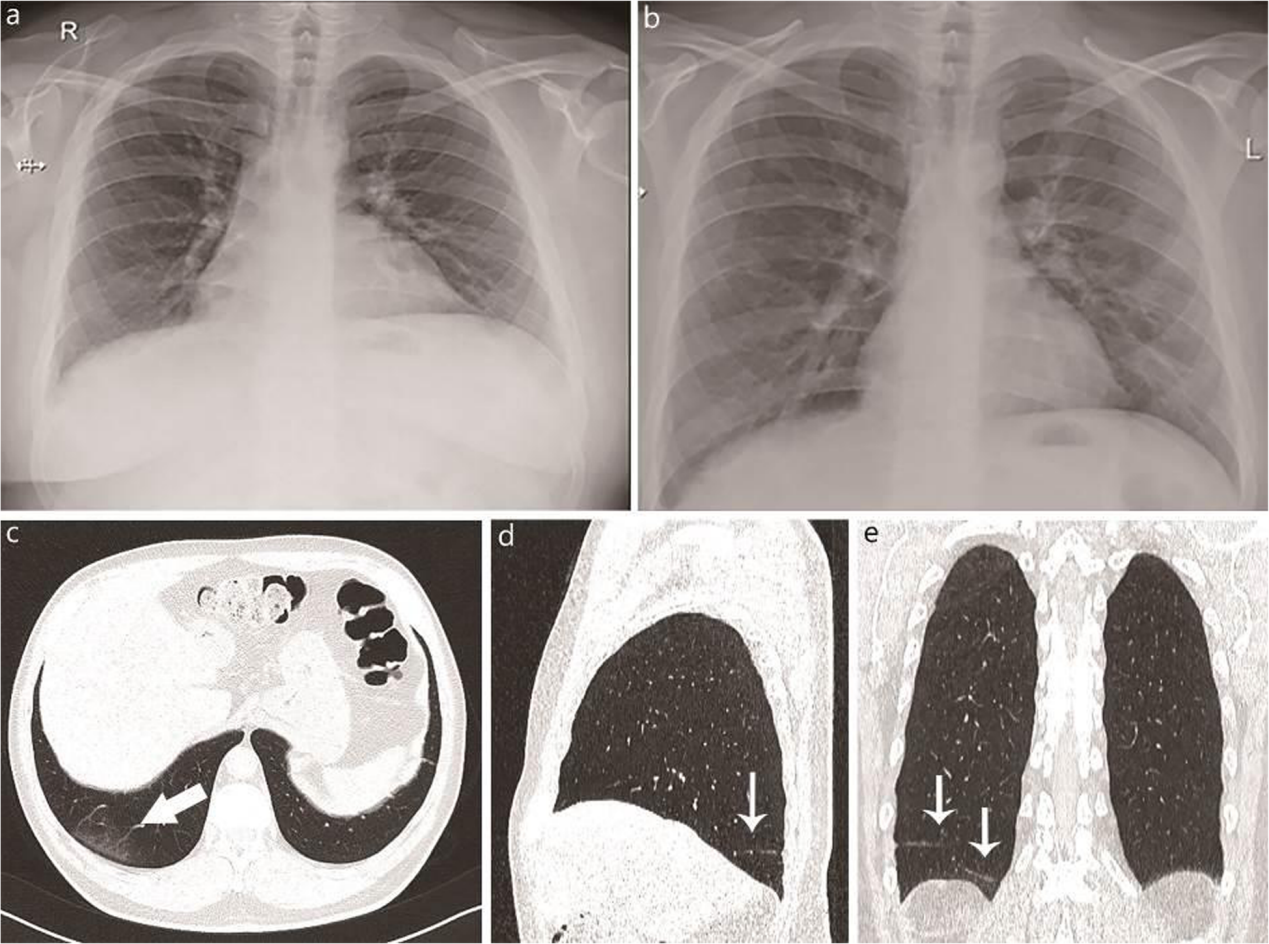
A 33-year-old male patient with band atelectasis mimicking GGO. Chest radiographs dated a. March 14, 2020 and b. March 22, 2020 are normal. c-e. CT images dated March 22, 2020 show focal patchy ground-glass density in the lower lobe of the right (thick arrow). Band atelectasis in axial sections, parenchymal distortions due to fibrotic disturbances may mimic the COVID-19 pattern (thin arrows). GGO. Ground glass opacity
